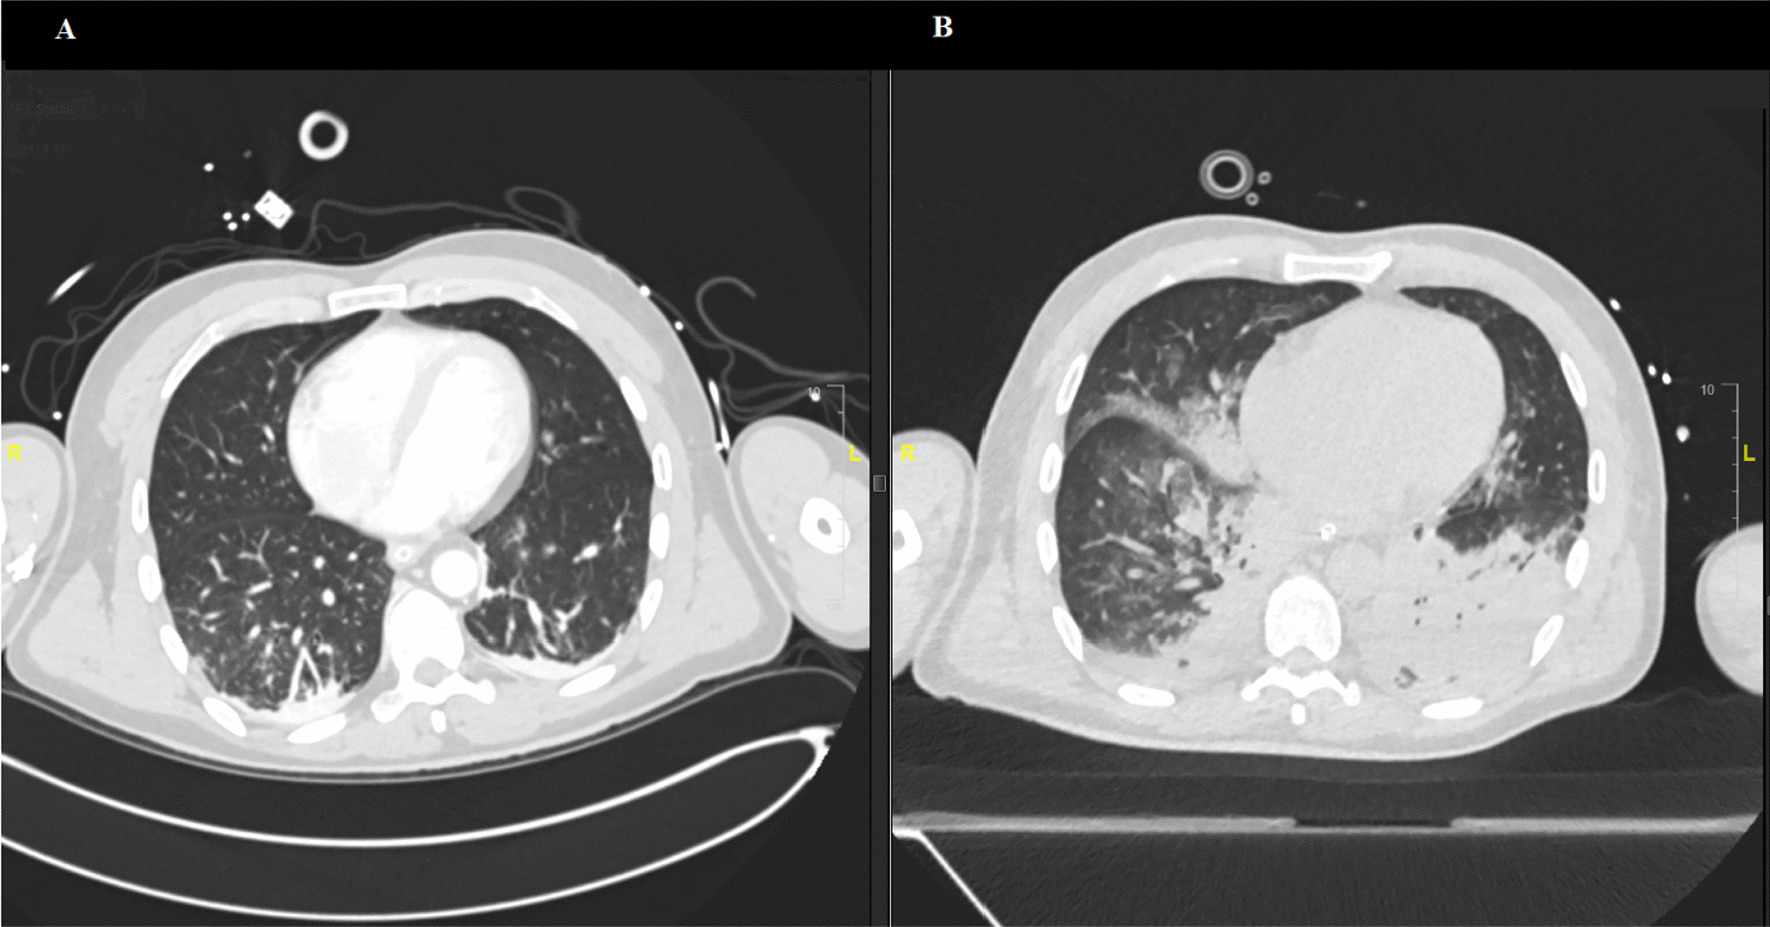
Computed tomography scans of the chest (A) on admission showing no signs of pulmonary infection (B) on the fifth day of stay in the ICU: patchy ground-glass opacifications and progressive consolidations typical for COVID-19 along with a small bilateral pleural effusions
